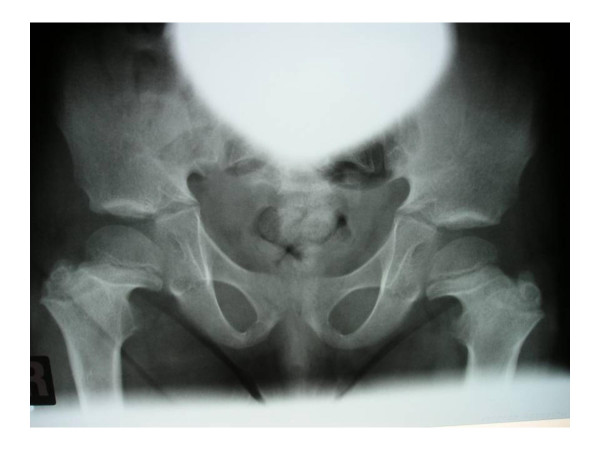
Anteroposterior radiograph of the pelvis showing rounded iliac bones, a horizontal acetabular roof and small sacroiliac notes. Coxa vara with defective modelling of the femoral necks associated with metaphyseal dysplasia with no trace of enchondromatous lesions.
